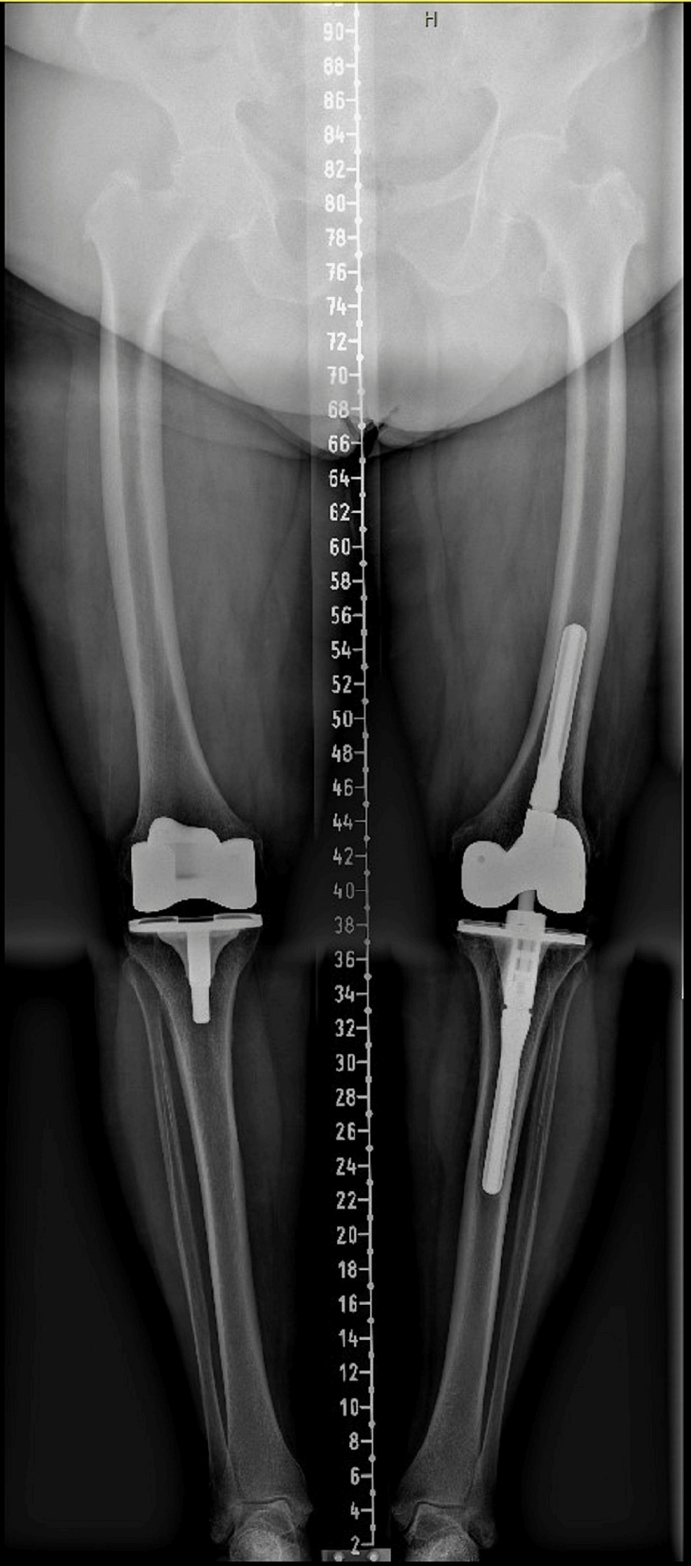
Full weight-bearing lower leg radiograph of the revision total knee arthroplasty previous to the haematogenous infection.
